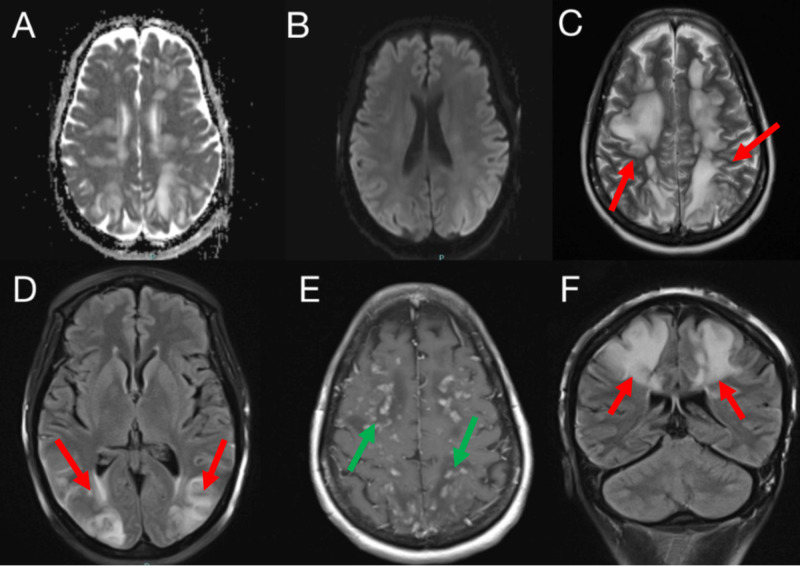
MRI of the brain with and without intravenous contrast: A (axial ADC), B (axial DWI), C (axial T2), D (axial T2 FLAIR), E (axial T1 with contrast) and F (coronal T2 FLAIR) Extensive confluent patchy areas of T2 and FLAIR hyperintensities involving the subcortical white matter of the bilateral frontal, parietal and occipital lobes (red arrows). Patchy foci of enhancement within the bilateral cerebral hemispheres (green arrows). No associated diffusion restriction ADC, apparent diffusion coefficient; DWI, diffusion-weighted imaging; FLAIR, fluid-attenuated inversion recovery
